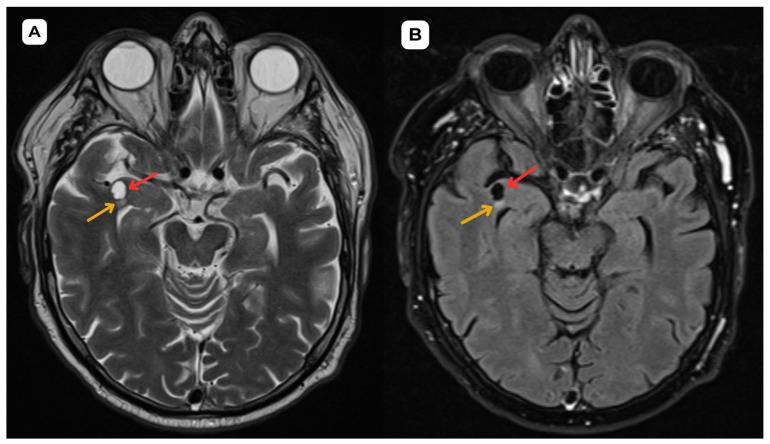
Accompanying second patient: One-year follow-up brain MRI of a 67-year-old male. (A) The T2-weighted sequence and (B) T2-FLAIR one-year follow-up MRI demonstrate no changes in the cystic lesion (red arrows) and surrounding structures. The minimal perifocal edema or gliosis (yellow arrows) has remained stable; no changes in size or signal intensity can be seen. These findings serve as an important criterion in uncertain cases of PVS; they are likely of a benign nature and consistent with an opercular (type IV) perivascular space. Red arrow—opercular (type IV) perivascular space; yellow arrow—surrounding edema. The stability of the lesion and surrounding tissue over the one-year period is reassuring. However, the need for continued monitoring in such cases remains a subject of discussion, as some studies suggest that these lesions tend to remain stable and do not require further follow-up unless there are significant changes [1]. For example, in a study by McArdle et al., 18 patients with opercular perivascular cysts were analyzed. Of the 13 patients who underwent follow-up over a period ranging from 2 months to 10 years, 11 showed no change in cyst size, and only one patient demonstrated a slight increase over a 7-month period [1]. These findings support the notion of long-term structural stability. Additionally, sources such as Radiopaedia.org highlight that opercular (type IV) perivascular spaces, when presenting with typical features, are considered benign and often do not require further imaging unless clinical symptoms or atypical imaging features are present. On the other hand, several authors suggest that such lesions, once identified correctly, typically require only follow-up imaging for monitoring [11,12]. Contrary to the generally stable nature of enlarged perivascular spaces over time, serial MRI studies show a prolonged increase in the size of low-grade neuroglial tumors, averaging 4.1 mm per year [13]. In this case, the aforementioned changes have remained stable over a one-year follow-up MRI. Literature on perivascular spaces, particularly type IV opercular perivascular spaces, is limited in documenting cases with or without surrounding vasogenic edema, and there are not many publications with detailed and structured radiologic images, especially zoomed-in images. This highlights the need for further publications that can provide a clear radiological depiction of such cases. Further studies and imaging examples are necessary to improve diagnostic accuracy and provide a comprehensive understanding of these benign structures.
